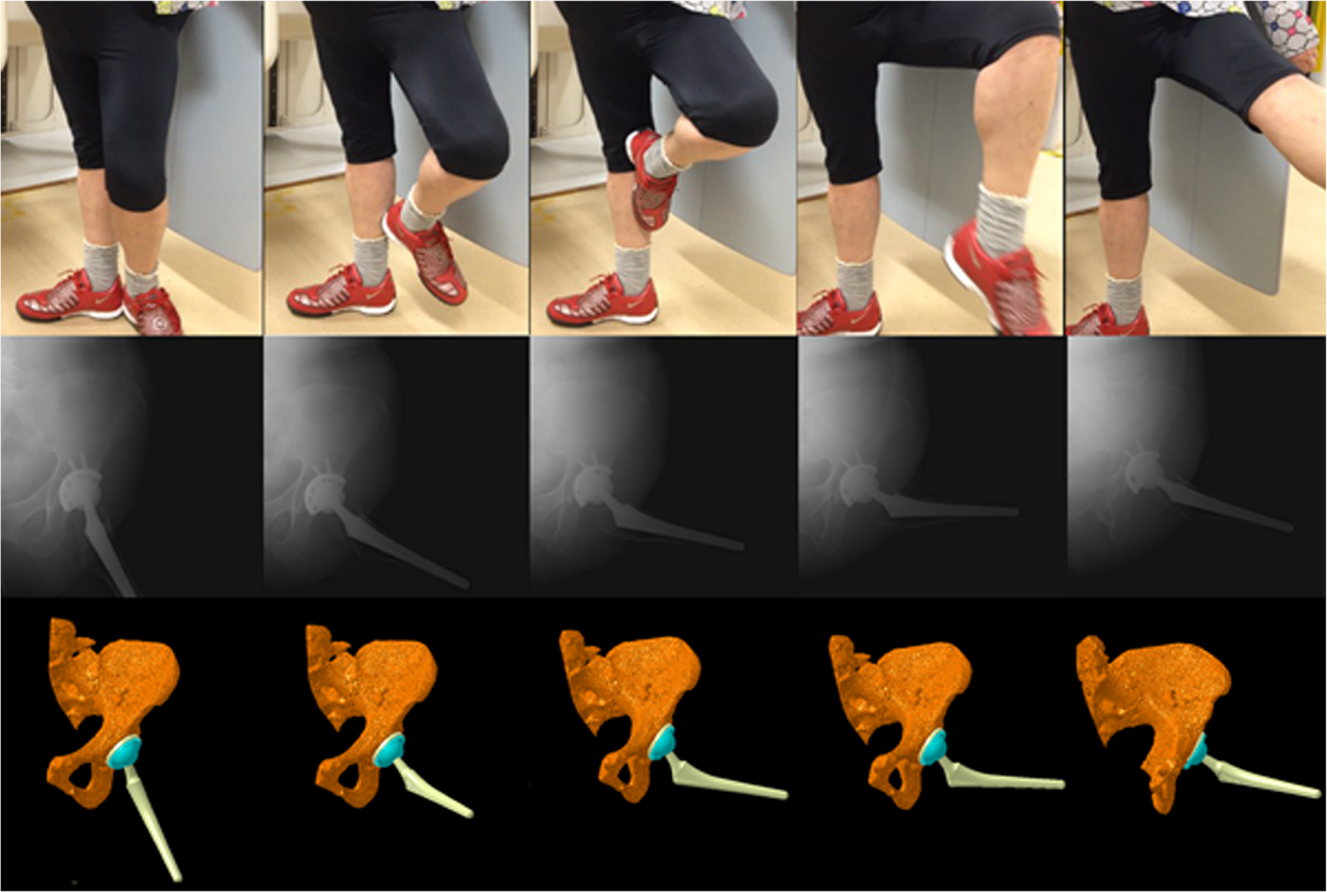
The hip motions during the ballet movement développé were captured as continuous X-ray images using a flat panel X-ray detector (*middle stand*) to reconstruct three-dimensional images of the replaced hip joint (*lower stand*) using image-matching techniques
